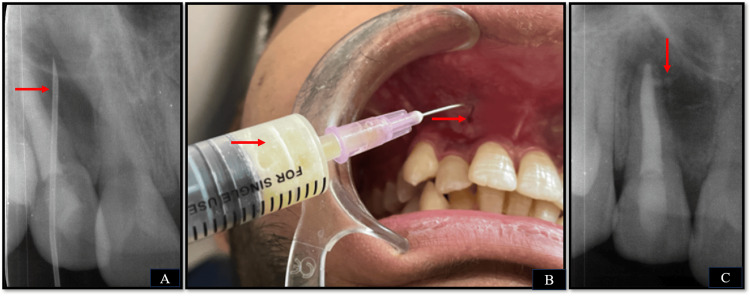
Extra-oral administration of combination of injectable platelet-rich fibrin and mineral trioxide aggregate (A) Radiographic confirmation of needle placement in the cystic cavity, (B) re-administration of the mixture in the periapical area through the buccal mucosa, and (C) radiograph confirming the placement of the mixture in the periapical area.
